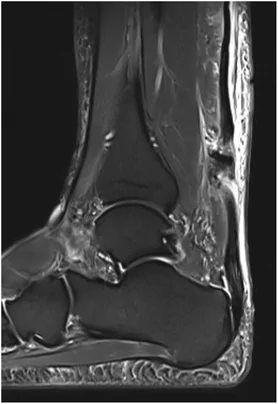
Preoperative supine T2‐weighted magnetic resonance imaging (MRI) of a left lower leg and foot showing a proximal Achilles tendon tear.
